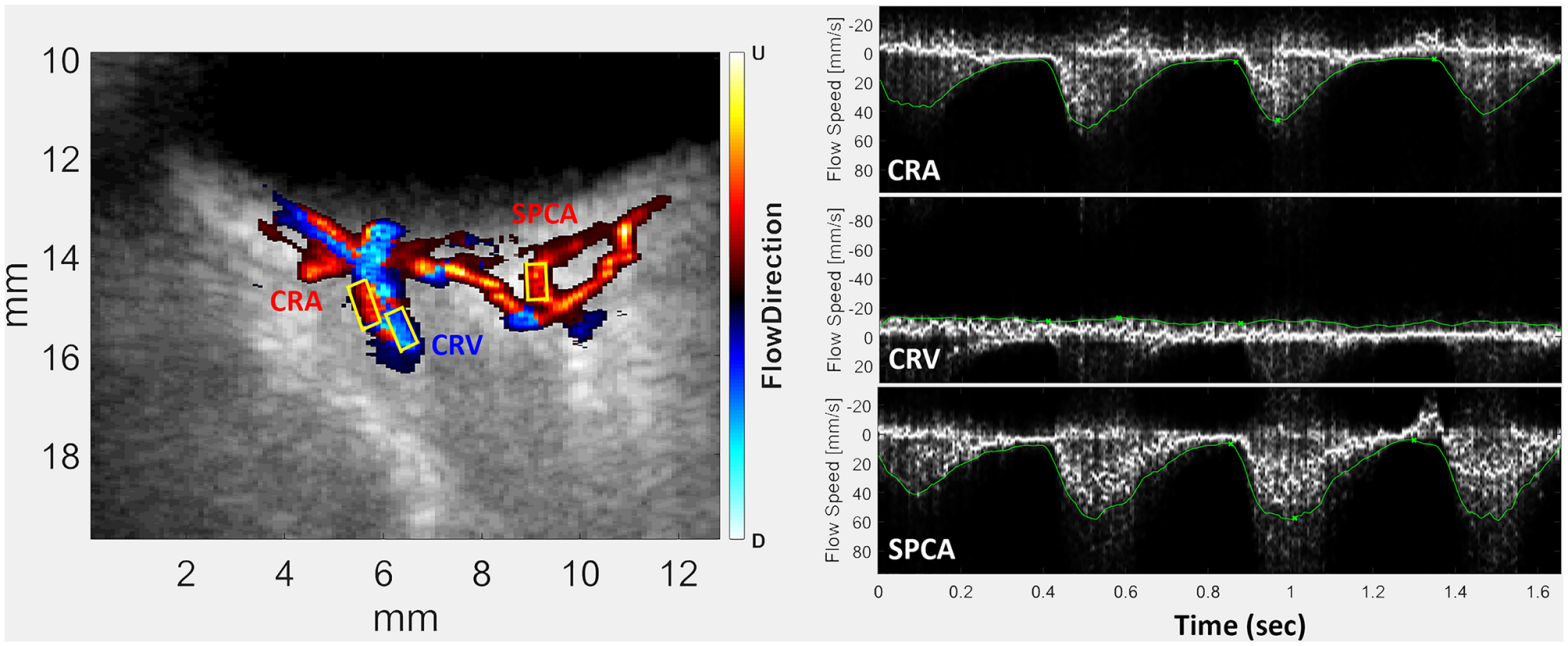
Left: Representative plane-wave color flow Doppler image of neonatal posterior pole. Right: Spectrograms of the CRA, CRV and SPCA.
